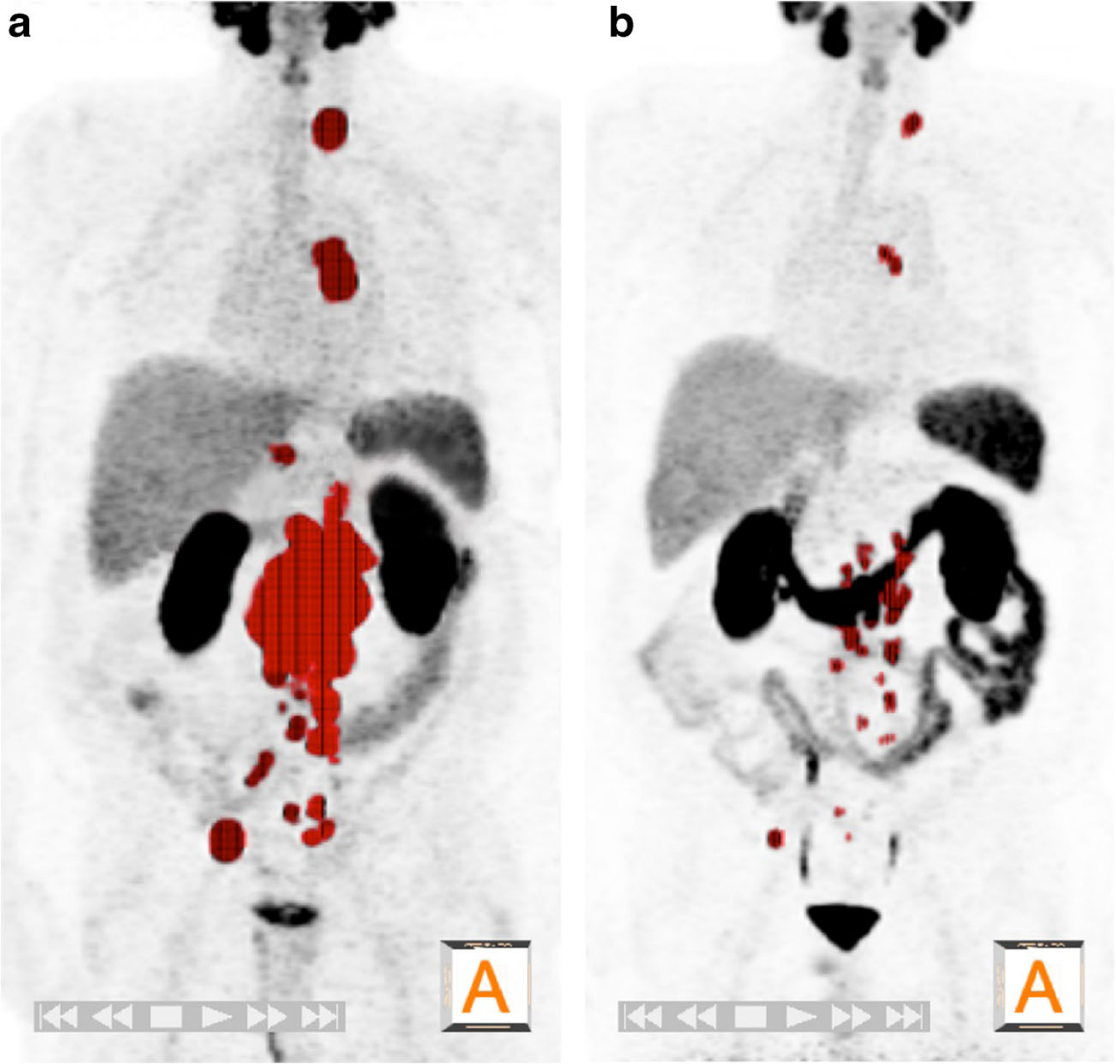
A 74-year-old patient with castration-resistant metastatic prostate cancer (mCRPC) after treatment with enzalutamide and docetaxel. a^68^Ga-PSMA 11 PET image before the first ^177^Lu-PSMA-617 radioligand therapy (RLT) in December 2015 demonstrates extended pelvic, abdominal and thoracic lymph node metastasis. The total tumor volume (TTV) measured semiautomatically as described in Materials and methods is marked *red* in the maximum intensity projection (MIP) 3D image. The PSA level at the time of imaging was 597 ng/ml, and the TTV was 359 ml. b After three cycles of RLT (7.4 GBq each), the PSA level had significantly decreased (23.1 ng/ml) and the TTV had reduced (43 ml), demonstrating a partial response. The patient received a further three cycles of RLT that resulted in a biochemical complete response (PSA 0.01 ng/ml, January 2018)
